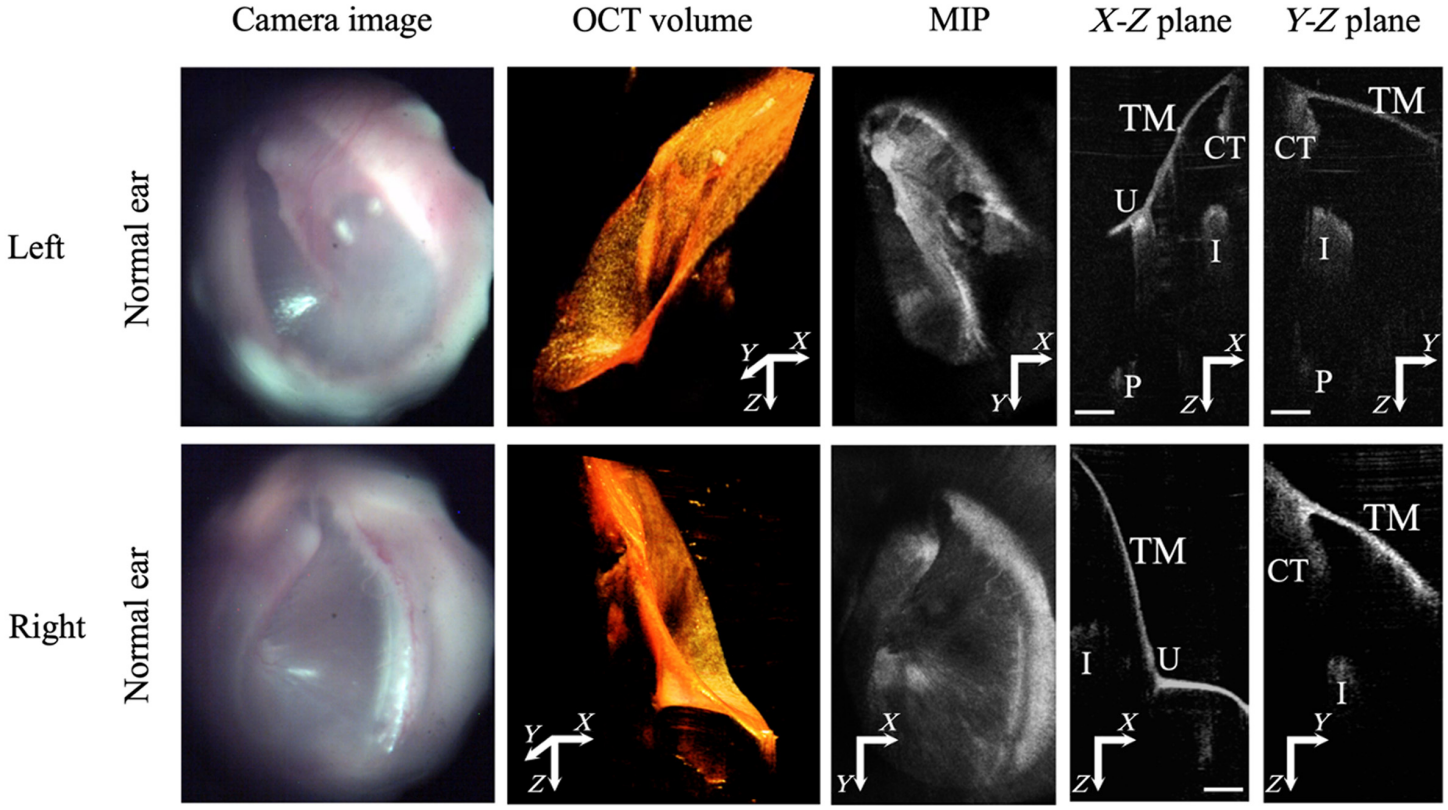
Otoscopic images and corresponding OCT volume images, mean intensity projection (MIP), and cross-sections, obtained from the OCT otoscope. Scale bar: 1 mm. CT: Chorda tympani. I: Incus. P: Promontory. U: Umbo. TM: Tympanic membrane.
